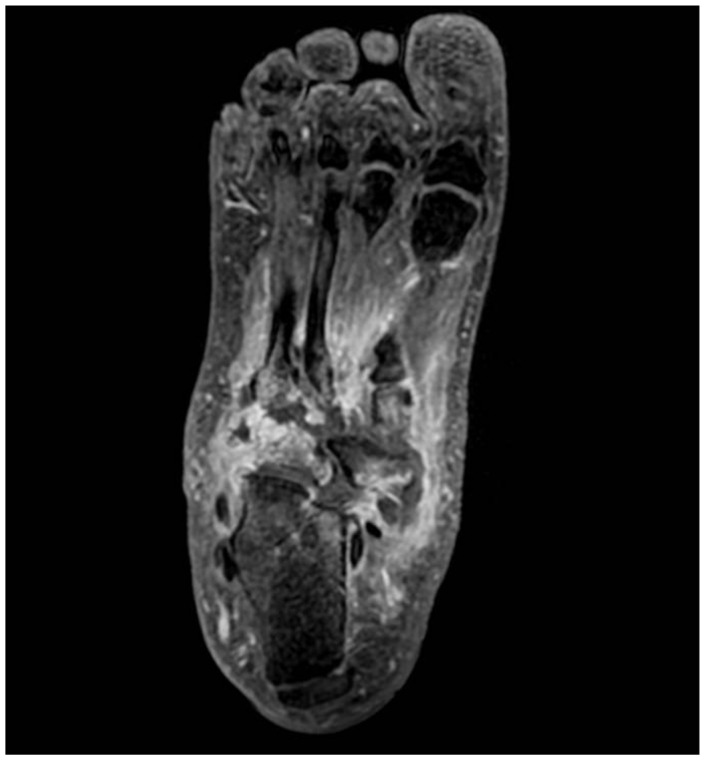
MRI (3D FSPGR + C): Subchondral bone marrow edema, joint effusions, and soft tissue inflammatory changes.
